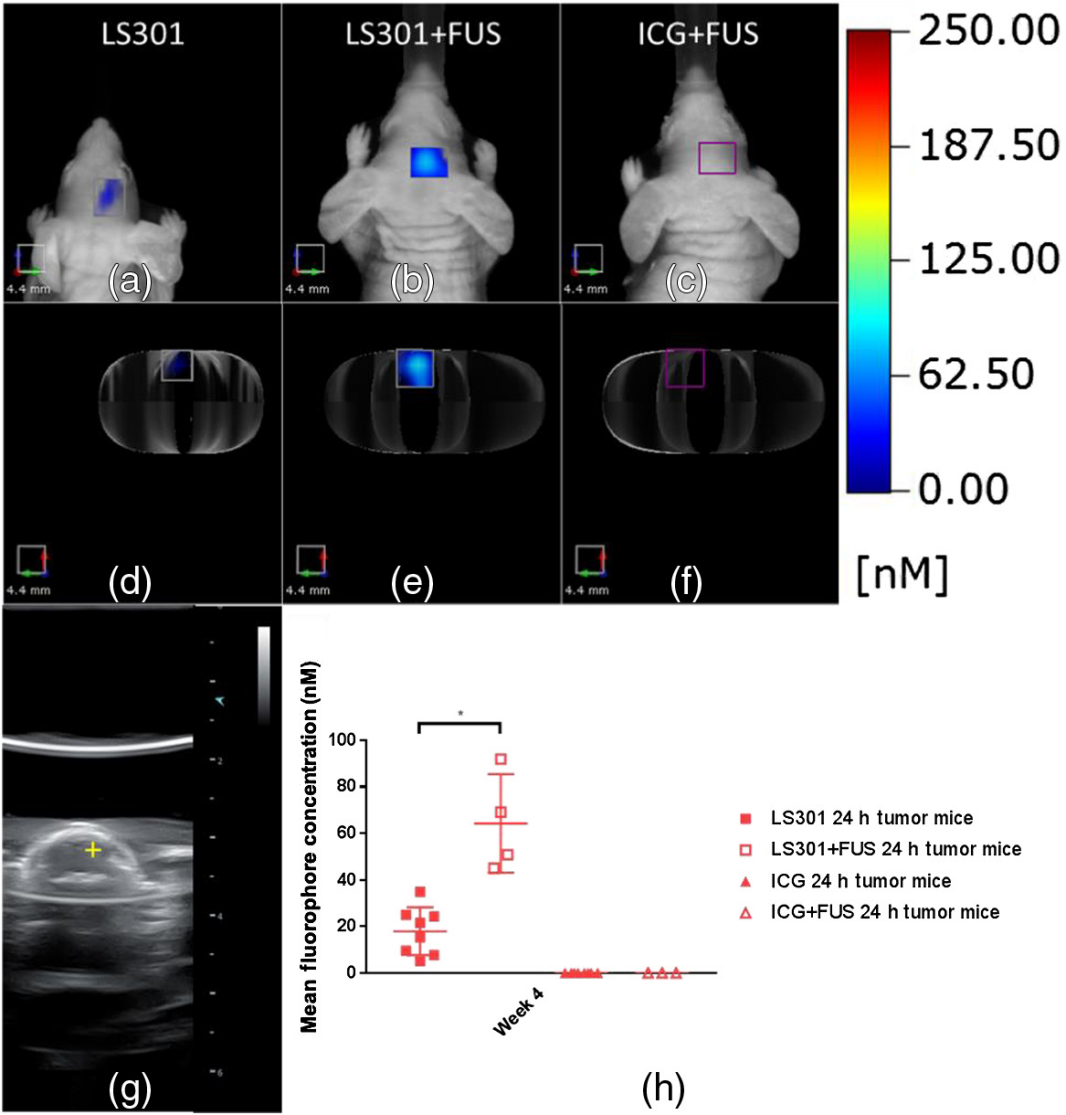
FUS delivery of LS301 and ICG at 4 weeks post-tumor initiation. (a), (d) Coronal and axial FMT projections of LS301 fluorescence at 24-h postinjection. (b), (e) Coronal and axial FMT projections of LS301 fluorescence at 24 h postinjection and FUS sonication. (c), (f) Coronal and axial FMT projections of ICG fluorescence at 24 h postinjection and FUS sonication. (g) B-mode ultrasound image showing focus of ultrasound treatment. (h) Quantification of tumor ROI fluorescence with and without FUS treatment.
